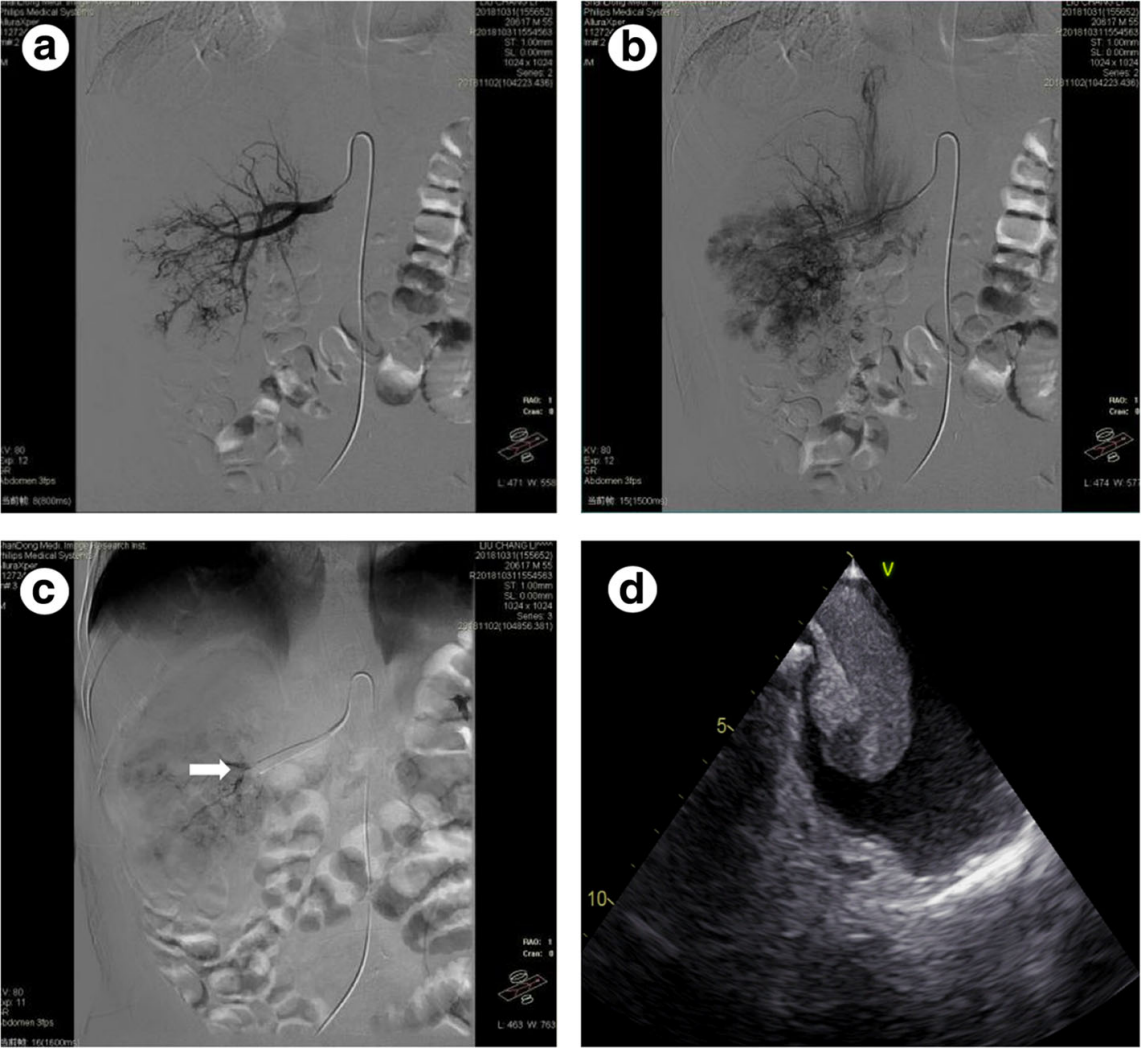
The renal arterial embolization of a 54-year-old renal cancer patient. a, b Renal arterial angiography demonstrating a right hypervascular renal cell carcinoma. c Performing the embolization of tumor vessels with gelatin sponge (white arrowhead). d The intraoperative trans-esophageal echocardiography showing the mobile thrombus mass extending across the tricuspid valve into the right ventricle
